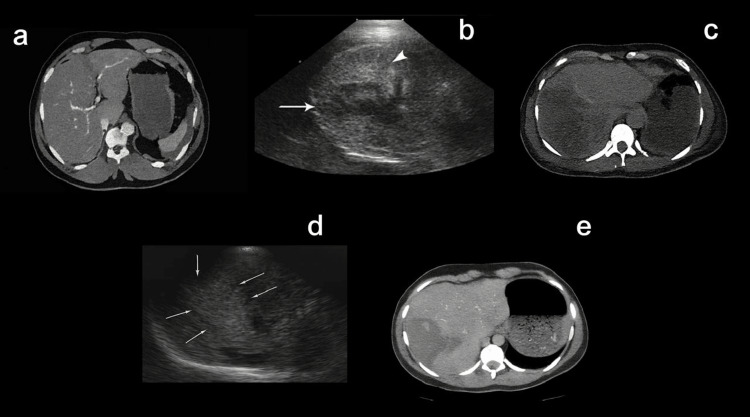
Imaging findings in liver trauma (a) Axial contrast-enhanced CT image showing a Grade II hepatic injury with a shallow parenchymal laceration and localized subcapsular hematoma. (b) USG image demonstrating a hypoechoic linear area (arrow) corresponding to a parenchymal laceration, with a focal hyperechoic area (arrowhead) suggestive of localized hematoma. (c) CT image showing a Grade III liver injury, characterized by a deeper laceration and associated perihepatic fluid collection. (d) Ultrasound image displaying multiple echogenic and hypoechoic areas (arrows) within the hepatic parenchyma, indicating heterogeneous echotexture and irregular margins consistent with parenchymal disruption and evolving hematoma formation. (e) Contrast-enhanced CT image revealing a large intraparenchymal hematoma with subcapsular extension, indicative of a Grade IV hepatic injury.
